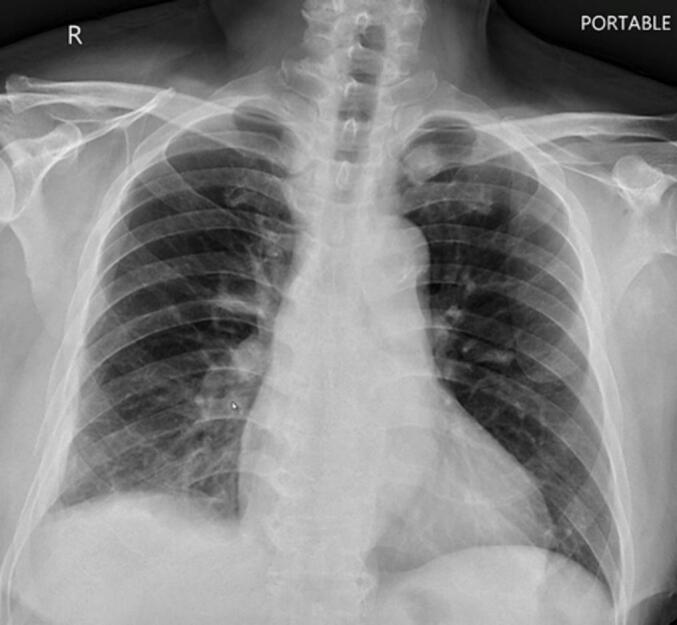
Patient's chest X-ray before discharge.
